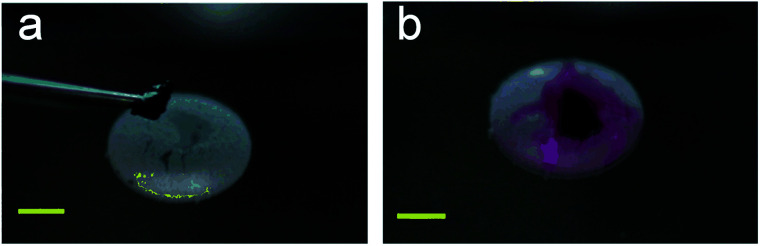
Levitation and operations of marbles by ultrasonics. The on–off motion of the LM’s shell is controlled by acoustic levitation. Reproduced from ref. 59 copyright 2015, American Chemical Society.
